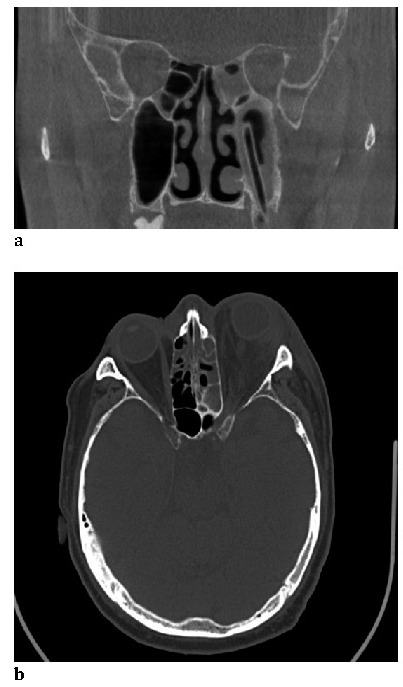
Sinus and orbital involvement on CT
